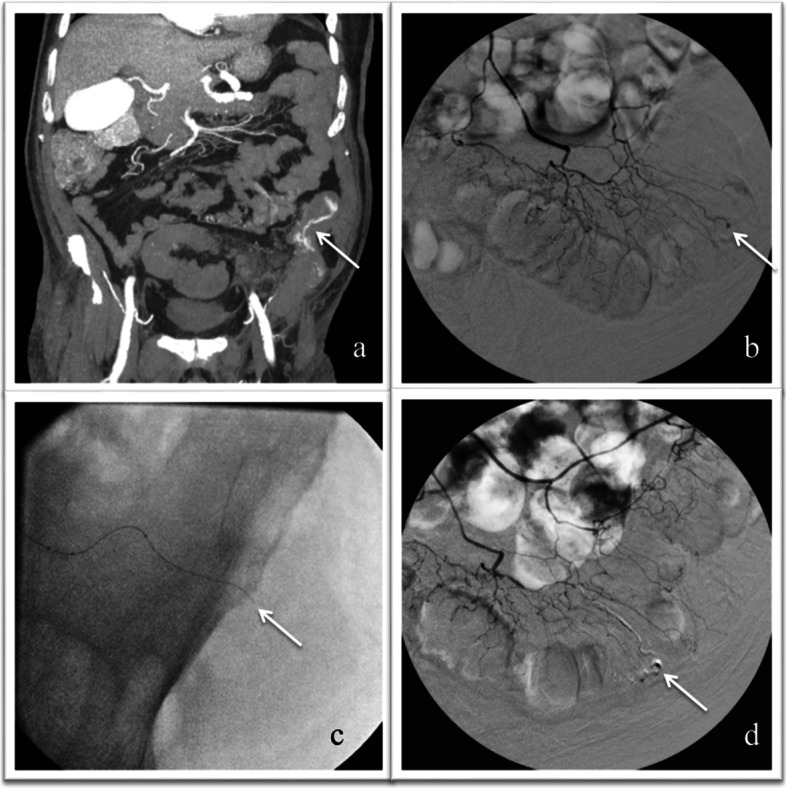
a–d CT MPR images in a patient suffering from lower gastrointestinal bleeding show active diverticular bleeding within the lumen of the descending colon (a). Selective inferior mesenteric artery angiography (IMA) shows a contrast extravasation from a branch of the sigmoid artery (b). After superselective catheterization of the target vessel with a 1.7 Fr microcatheter (c), selective IMA post-embolization angiography confirms selective occlusion of the target vessel (d)
